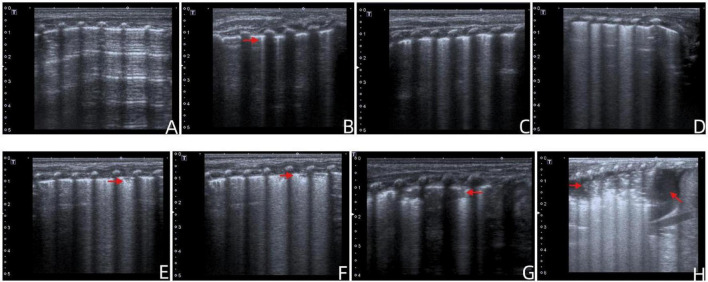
Representative lung ultrasound images for each category of the modified twelve-zone LUS score (0–7 points). (A) Female neonate, 13 days old. Predominantly A-lines with fewer than three isolated B-lines (score 0). (B) Male neonate, 10 days old. Right anterior upper lung zone with more than three non-confluent B-lines (score 1). (C) Male neonate, 21 days old. Left upper lung zone showing dense, partially fused B-lines (score 2). (D) Female neonate, 11 days old. Right lower lung zone with completely fused B-lines (score 3). (E) Male neonate, 19 days old. Right anterior lower lung zone with a small subpleural consolidation involving less than one intercostal space (score 4). (F) Female neonate, 22 days old. Left lower lung zone with consolidation involving 1–2 intercostal spaces (score 5). (G) Female neonate, 16 days old. Left posterior lower lung zone with consolidation involving 2–3 intercostal spaces (score 6). (H) Male neonate, 17 days old. Right posterior lower lung zone with consolidation involving more than three intercostal spaces accompanied by pleural effusion (score 7).
